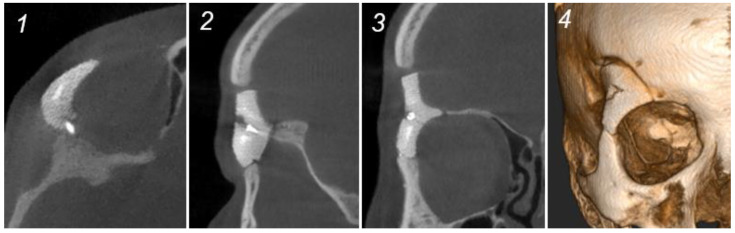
Radiological (CBCT) and clinical situation after 6 months, case 1. (1): Axial plane. (2): Sagittal plane—signs of osteogenesis. (3): Frontal plane—signs of osteogenesis. (4): 3D CT image.
